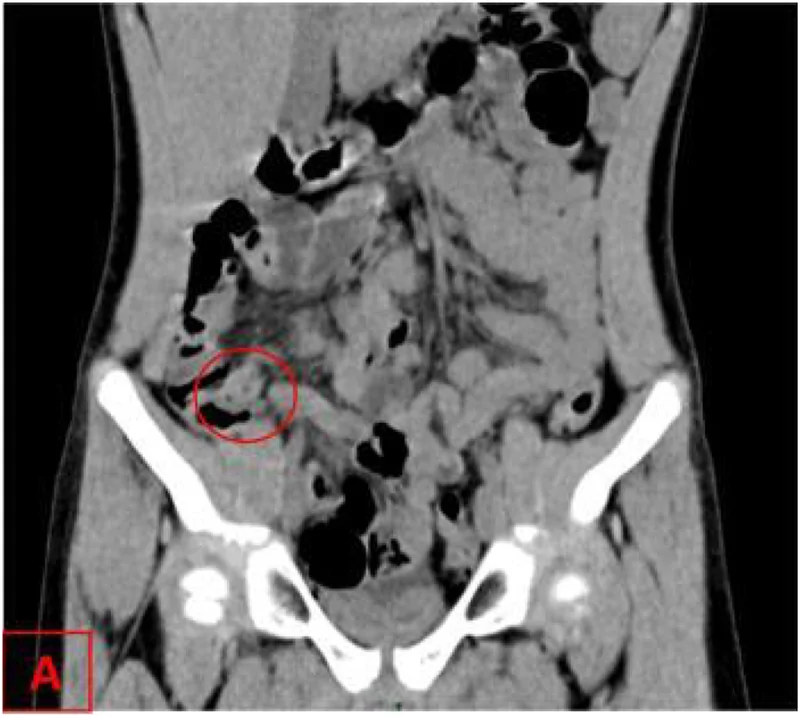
Preoperative contrast-enhanced computed tomography images. (A) Axial CT image demonstrating mild appendiceal dilatation (approximately 7 mm in diameter) with surrounding fat stranding (red circle), mild ileocecal wall thickening, and a small amount of pelvic free fluid, consistent with acute appendicitis.
